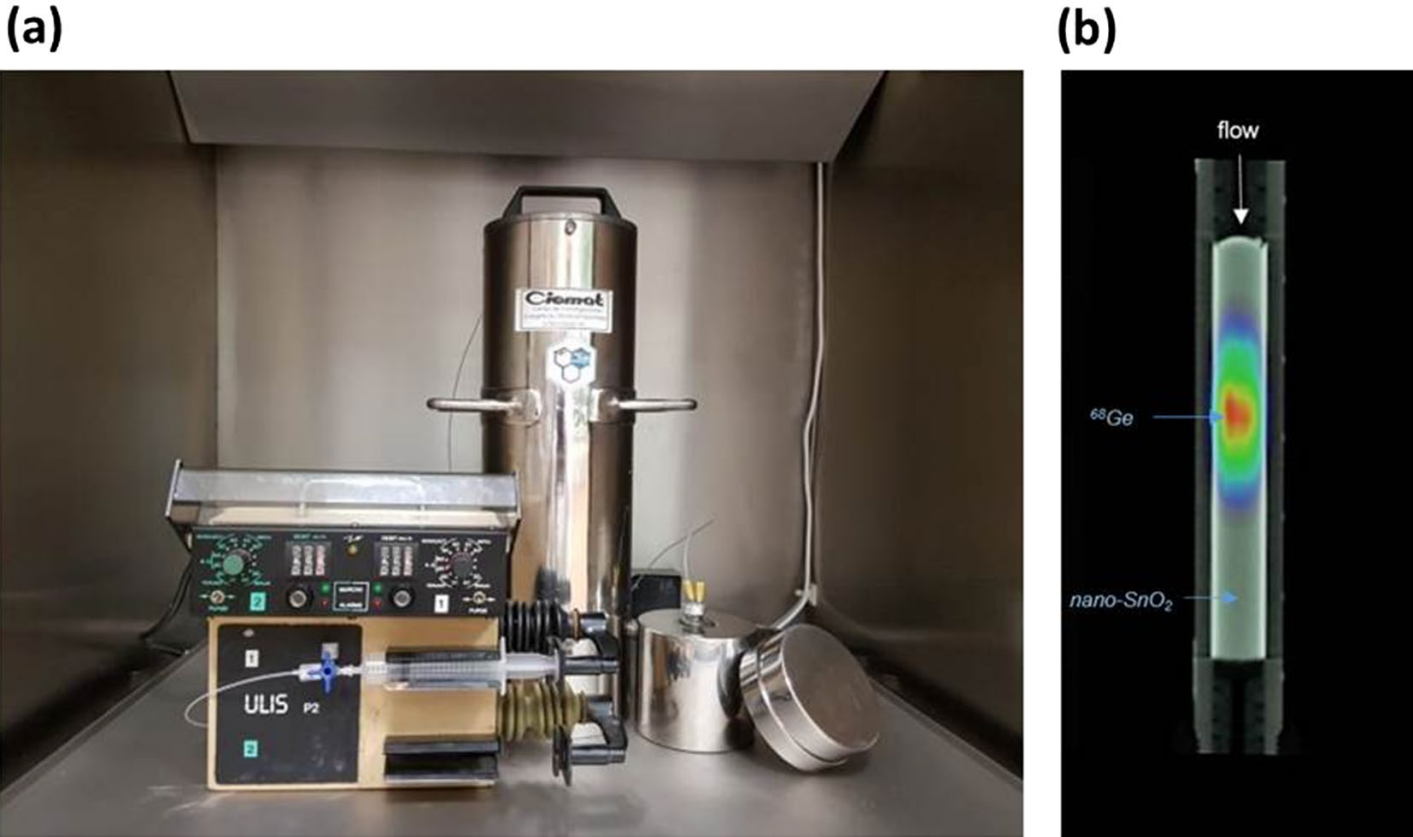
^68^Ge/^68^Ga generator. (a) The column was maintained in an easily-transportable lead shielded cylindrical shape. (b) Sagittal view PET/CT image of generator column after of 17 months (305 elution cycles).
